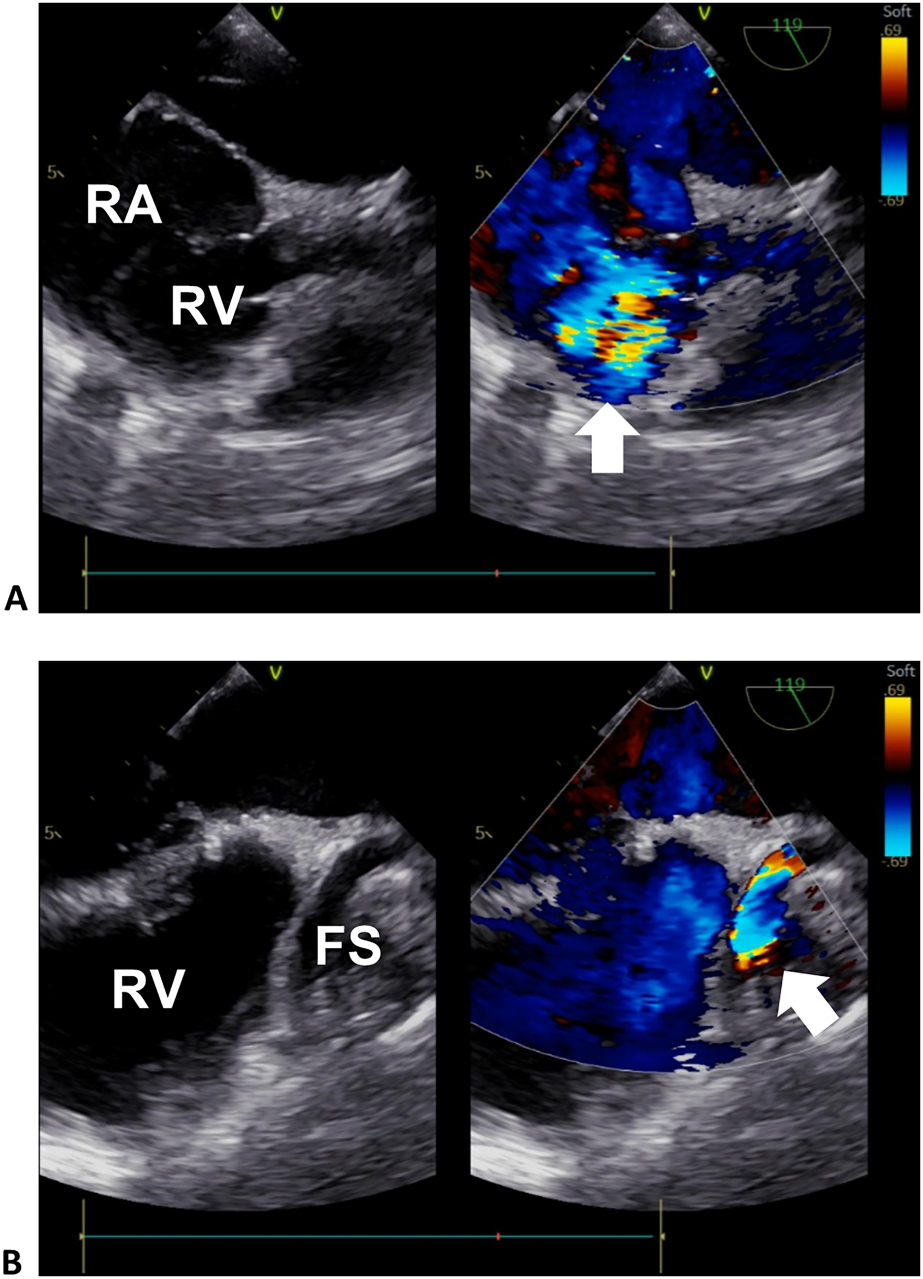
Transesophageal echocardiogram of the right heart. (A) Sectional plane showing the exit flow from the fistula sac into the right heart. (B) Sectional plane showing the entrance flow from the RCA to the fistula sac. The arrows denote the turbulence signals of the exit and entrance flows in (A) and (B), respectively. RA, right atrium; RV, right ventricle; FS, fistula sac.
